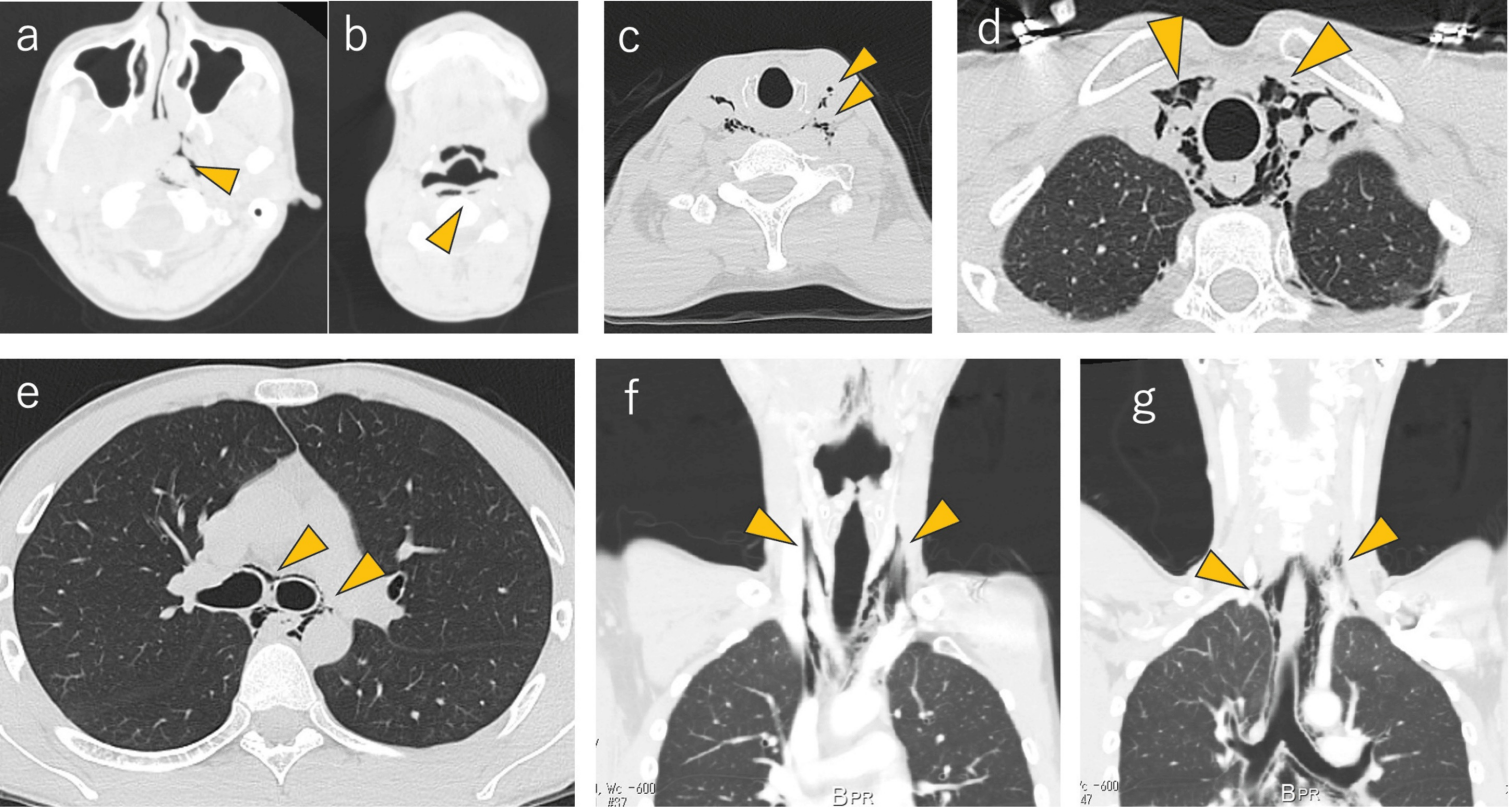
Images of emphysema on computed tomography A plain CT revealed the presence of emphysema (arrowheads) from the nasopharynx to the left parapharyngeal space (a), retropharyngeal space (b), both sides of carotid artery space (c.d), and mediastinum (e). Images (f) and (g) show the coronal view of the emphysema.
